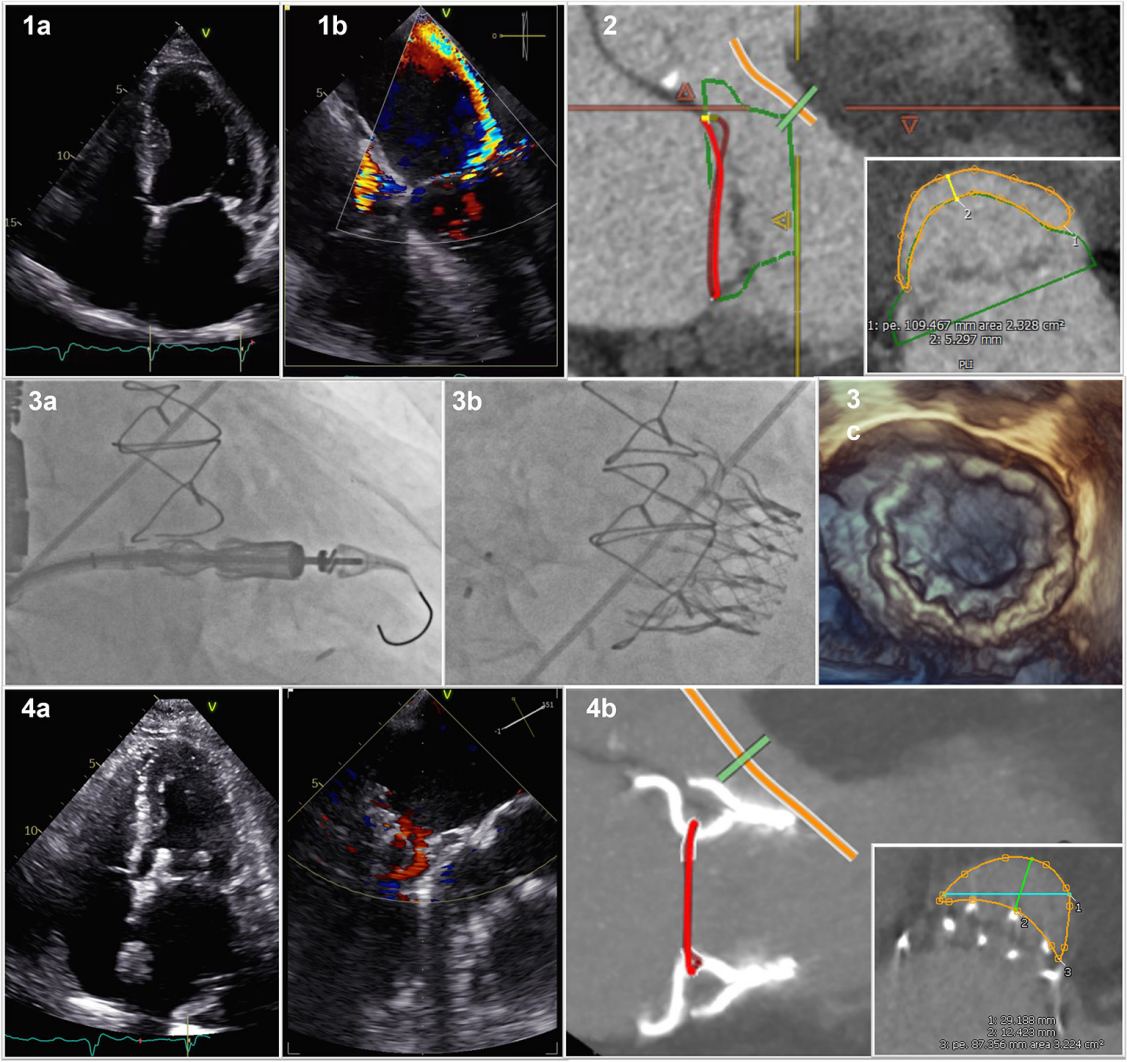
Cardiovalve in Mitral Valve Position. (1a) 2D posterior mitral leaflet by calcification; (1b) consecutive severe excentric posterior jet; (2) Pre-procedural computed tomography scan with valve sizing and predicted neo-LVOT; (3a) Fluoroscopy of device delivery; (3b) Fluoroscopy of device with closure of ASD; (3c) 3D transesophageal echocardiography of delivered Device; (4a) post-interventional result; (4b) Post-procedural CT scan with actual neo-LVOT.
